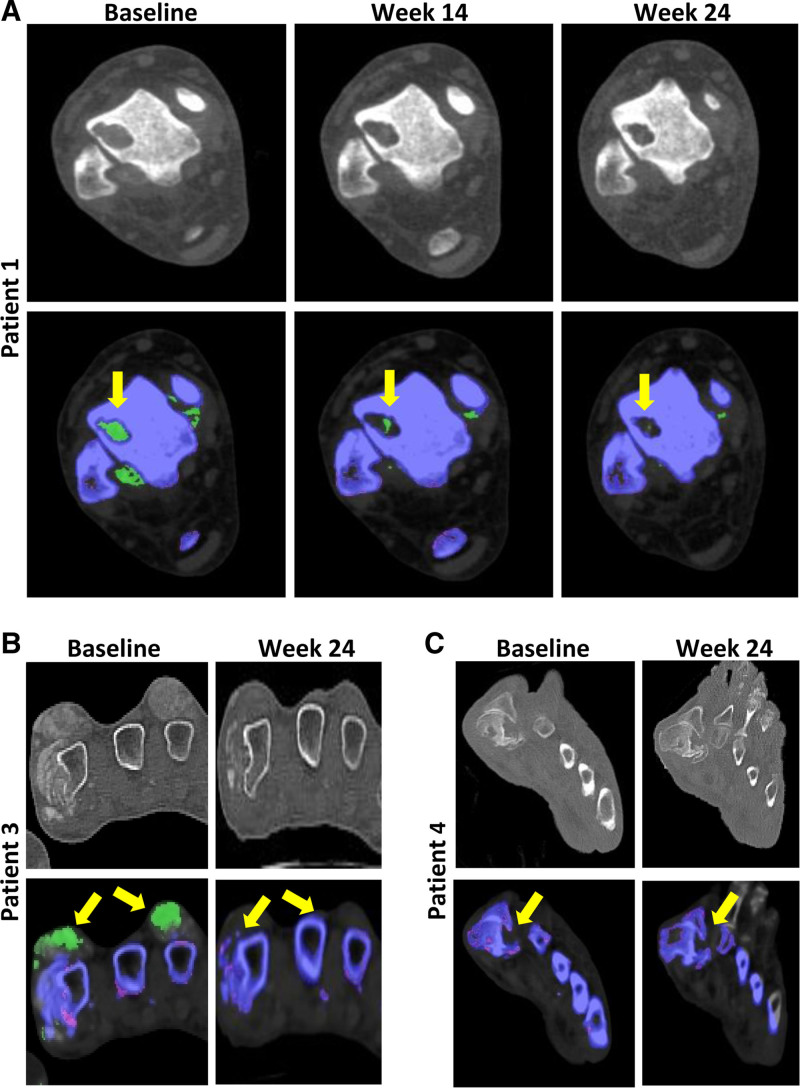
Serial DECT images during pegloticase treatment showing the 3 types of observed erosions: adjacent to urate (A; axial CT, talus of the ankle), adjacent to both color-coded MSU and other mineralized matrix (B; axial CT, metacarpals of hand), and not adjacent to a color-coded MSU deposit (C; long axis CT, first metatarsal). Concomitant with urate deposit depletion, the urate-adjacent erosion had decreased in size by week 24 in this patient without radiologic features of CKD-MBD (A). No changes in the other erosion types were observed in patients with radiologic features of MBD (B and C). Urate deposition is represented in green. Other mineralized deposition is represented in white/purple. CKD-MBD, chronic kidney disease-mineral and bone disorder; CT, computed tomography; DECT, dual-energy CT; MSU, monosodium urate.
